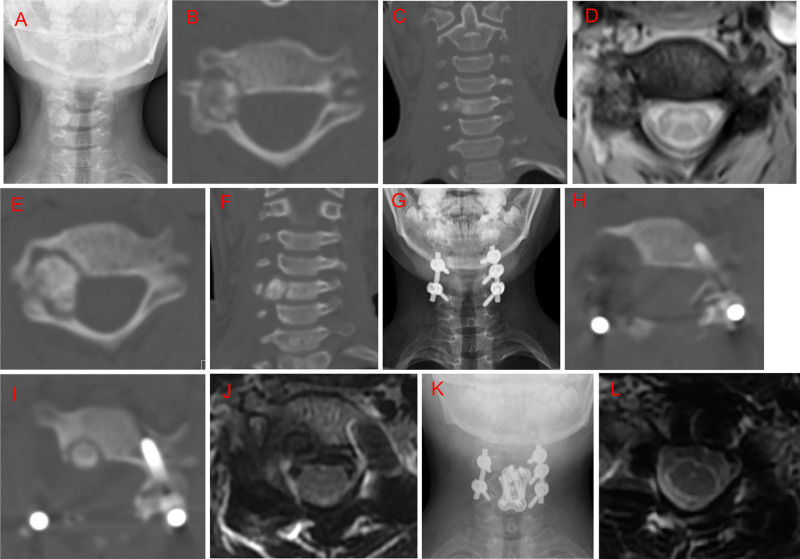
Case 6. A 10-year-old female patient with a history of neck pain over 6 months. An irregular and heterogeneous lesion was located in the C5 right pedicel and transverse process, vertebral body, and lamina, with right vertebral artery extension. She initially underwent radiofrequency ablation; however, computed tomography (CT) examination at the 5-month follow-up revealed lesion enlargement. Subsequently, the patient underwent intralesional marginal resection through a posterior approach. Local recurrence was identified by CT at the 12-month follow-up. She underwent a C5 vertebrectomy through an anterior approach. No recurrence was observed at the 21-month follow up after the second surgery. (A) Posteroanterior radiography before radiofrequency ablation. (B,C) Preoperative axial and coronal CT scans before radiofrequency ablation. (D) T2-weighted magnetic resonance imaging (MRI) scan at 5 months after radiofrequency ablation. (E,F) Axial and coronary CT scan at 5 months after radiofrequency ablation. (G) Posteroanterior radiography 1 week postoperatively. (H) Axial CT scan 1 week postoperatively. (I) Axial CT scan 12 months postoperatively. (J) T2-weighted MRI scan 12 months postoperatively. (K) Posteroanterior radiography 1 week after the second surgery. (L) T2-weighted MRI CT scan 21 months after the second surgery.
